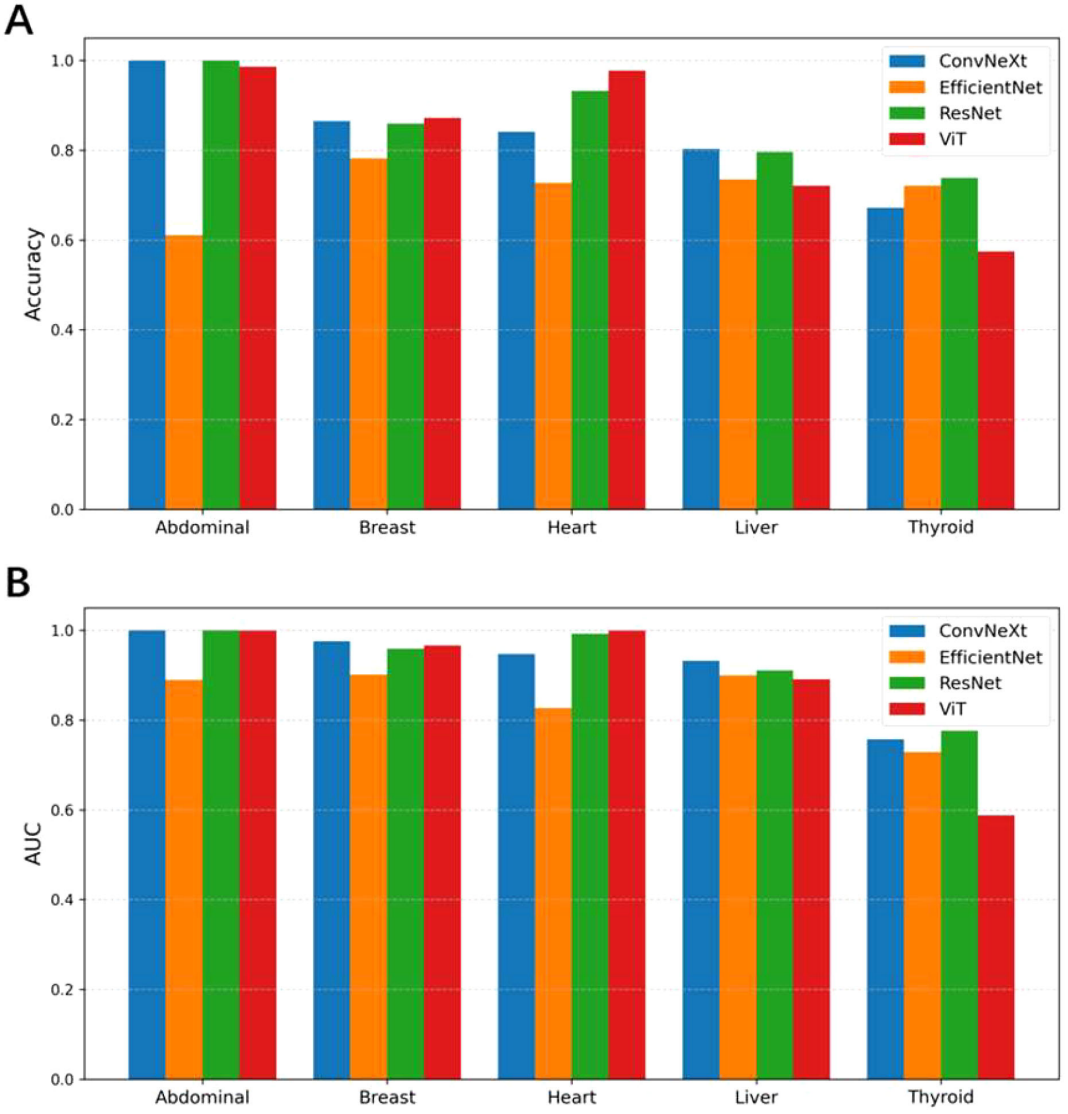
Comparison of classification accuracy (A) and AUC values (B) among different models (ConvNeXt, EfficientNet, ResNet, and ViT) across five pretrain ultrasound tasks (Abdominal, Breast, Heart, Liver, and Thyroid). Each group of bars represents the models’ performance on a specific task.
